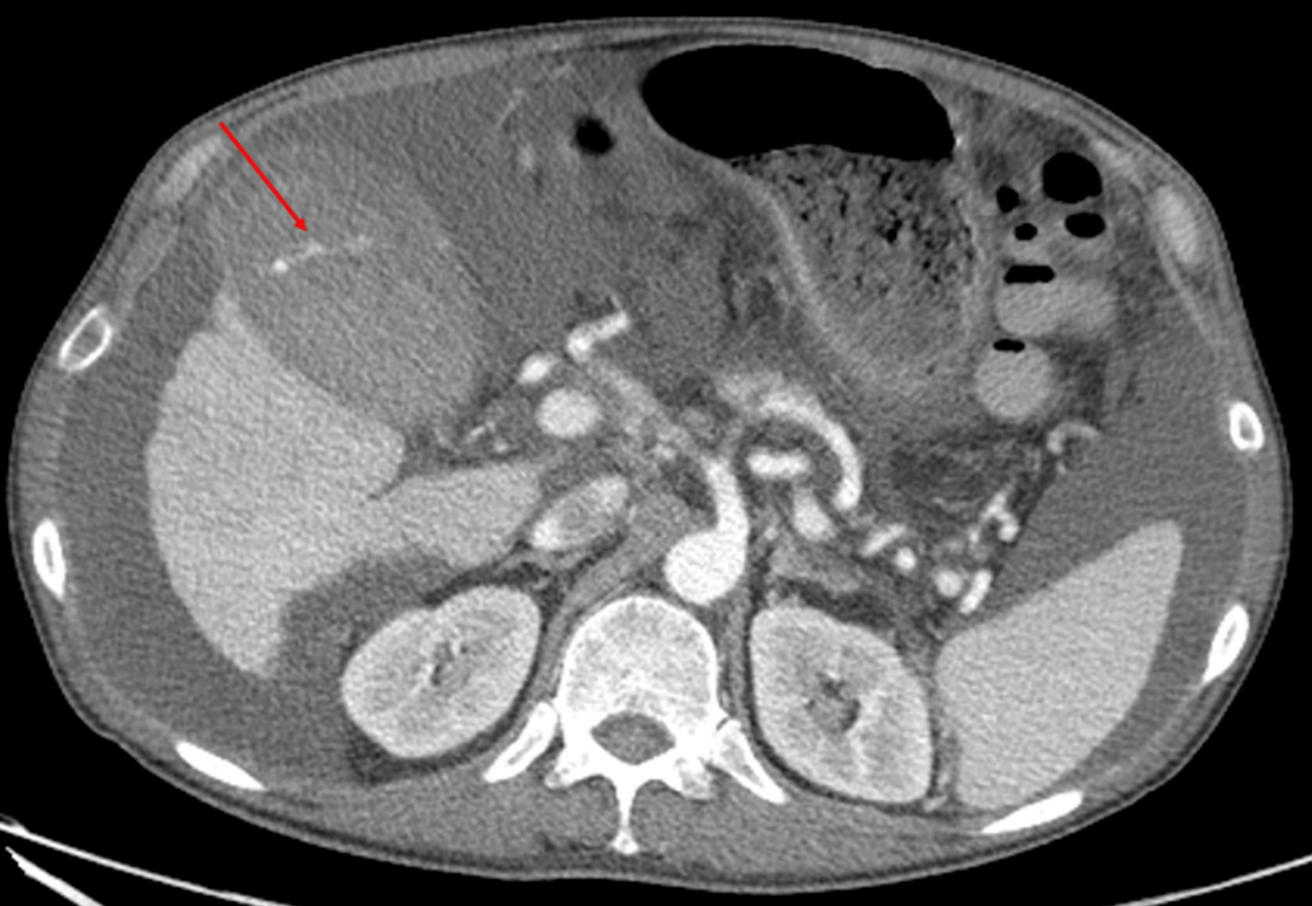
Axial computed tomography (CT) imaging with intravenous contrast in the arterial phase. Imaging obtained after the patient developed abdominal pain and acute anemia post-transjugular liver biopsy shows active contrast extravasation into the gallbladder lumen (red arrow).
